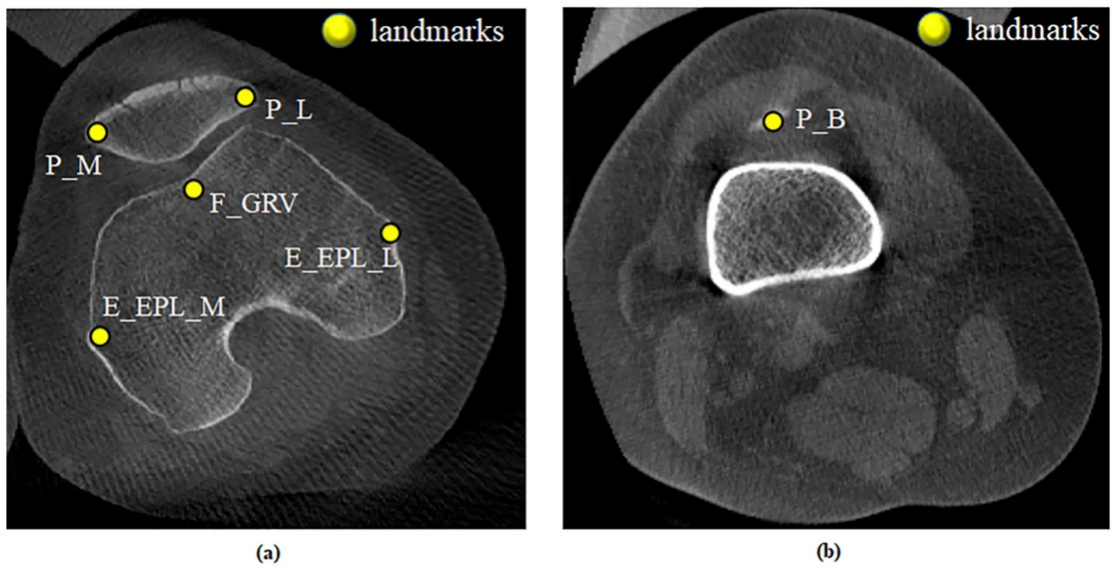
Representative tracking points on CT images. (a) Landmarks on the patella and femur. (b) Landmark on the patella. Abbreviations: P_L, patella lateral; P_M, patella medial; P_B, patella bottom; E_EPL_L, femur lateral; E_EPL_M, femur medial; F_GRV, femur groove.
